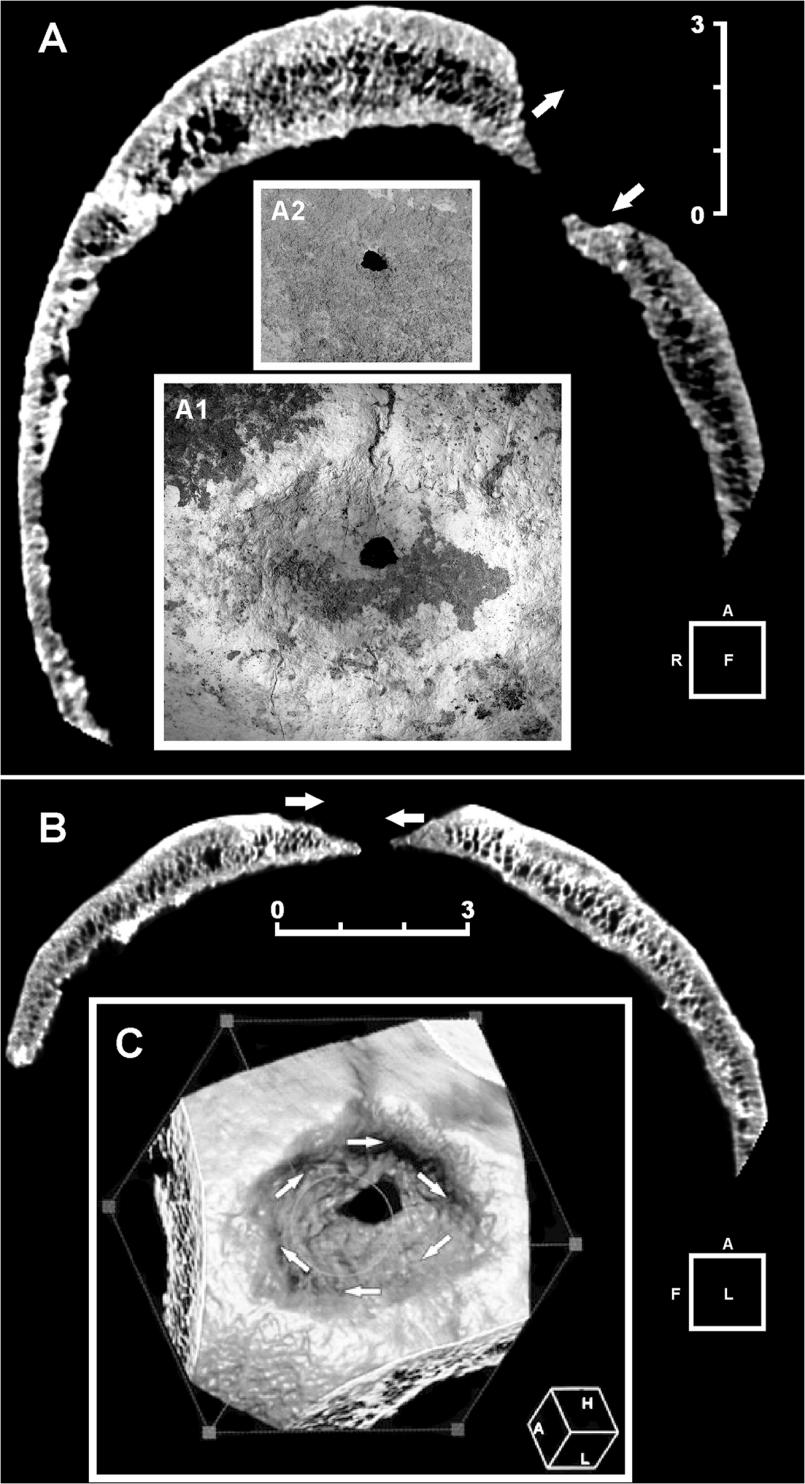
Three-dimensional (3D) computed tomography (CT) scan of the main skull trephination. Frontal (A) and sagittal (B) plane CT scan. Extensive and irregular bone reconstruction is apparent. No evidence is seen of incomplete healing processes as in the case of complication by infection. Note the different angle at the top/bottom (frontal plane) (A) and front/back (sagittal plane) (B) bone around the hole, indicative of right-handed clockwise rotation applied during the action of scraping (arrows). Detail of the main lesion, whose edges show active regenerative bone processes (A1). Endocranial aspect of the lesion. The bone surrounding the hole is intact (A2). 3D reconstruction of the outer aspect of the trepanned cranial vault. The arrows show the clockwise rotation movement (C). Scale bars measure 3 cm.
